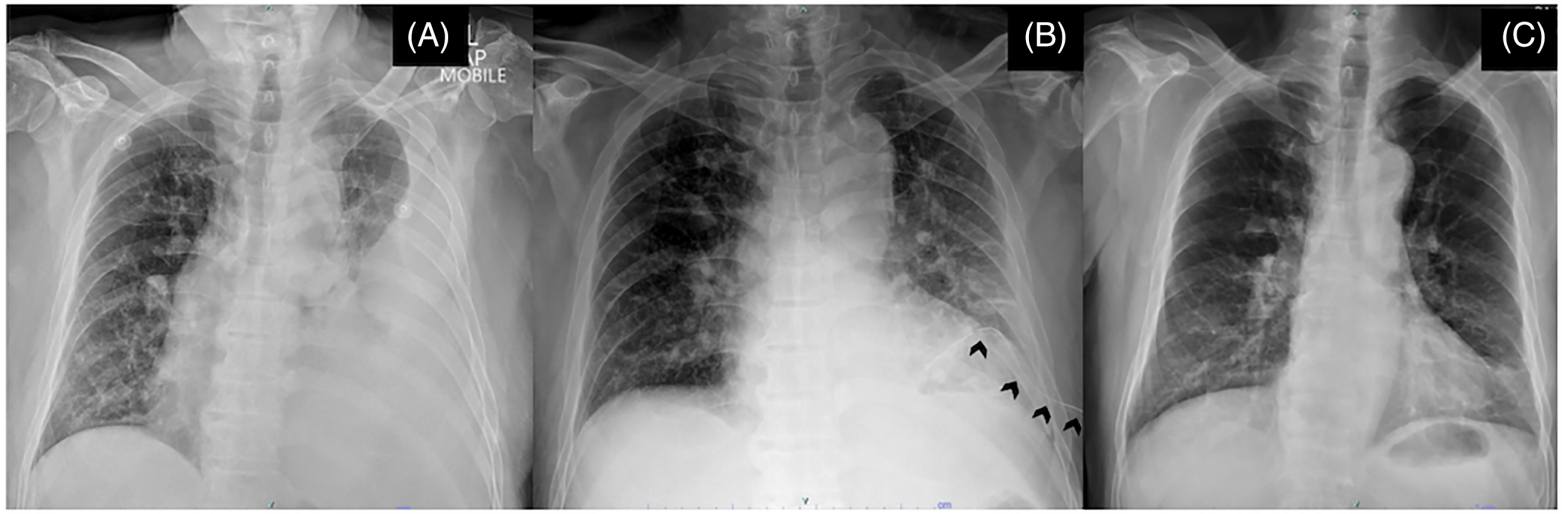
(A) Chest radiograph showed a moderate pleural effusion. (B) Chest radiograph after completion of three cycles of intrapleural saline irrigation and intrapleural tyloxapol showed significant effusion of left pleural effusion; the ICC (arrow) was in‐situ. (C) Chest radiograph on follow‐up 6 weeks later showed resolution of pleural effusion.
